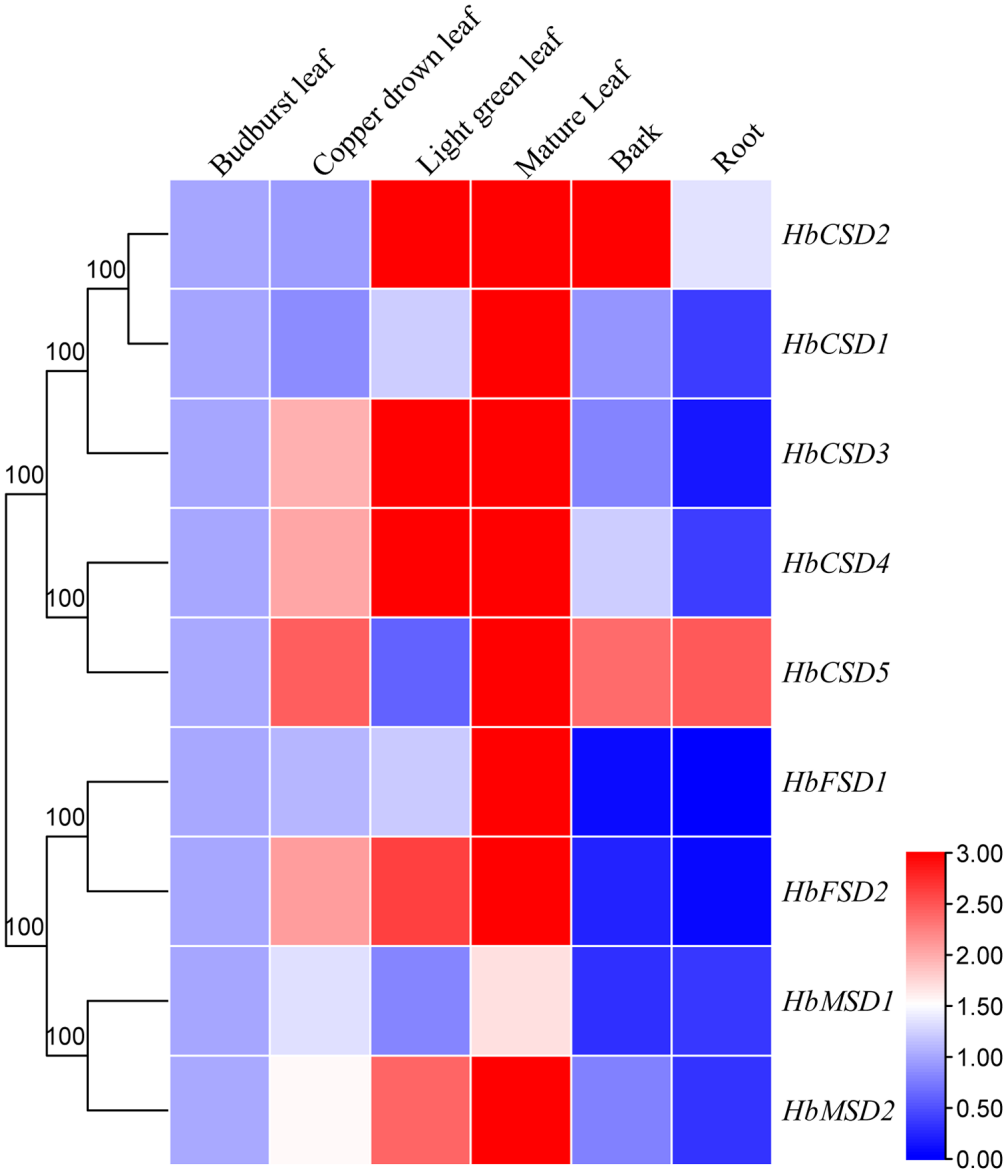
Expression analysis of *HbSOD* genes in several organs. The expression levels were measured with 2^−∆∆CT^ and treated as double normalized using the reference genes and the expression at the bud-burst leaf.
